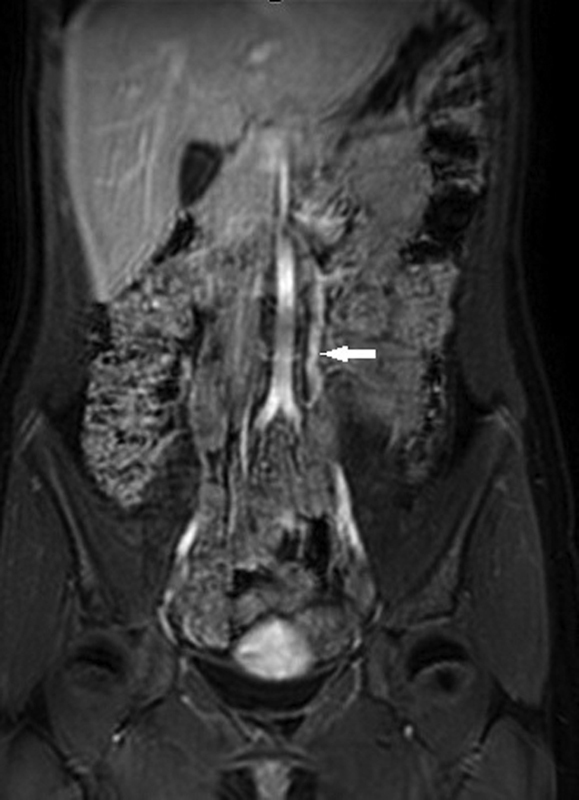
Magnetic resonance imaging showing a dilated left ovarian vein (white arrow) with a caliber of 8 mm and a grade II reflux, draining into the retroaortic component of the left renal vein.
